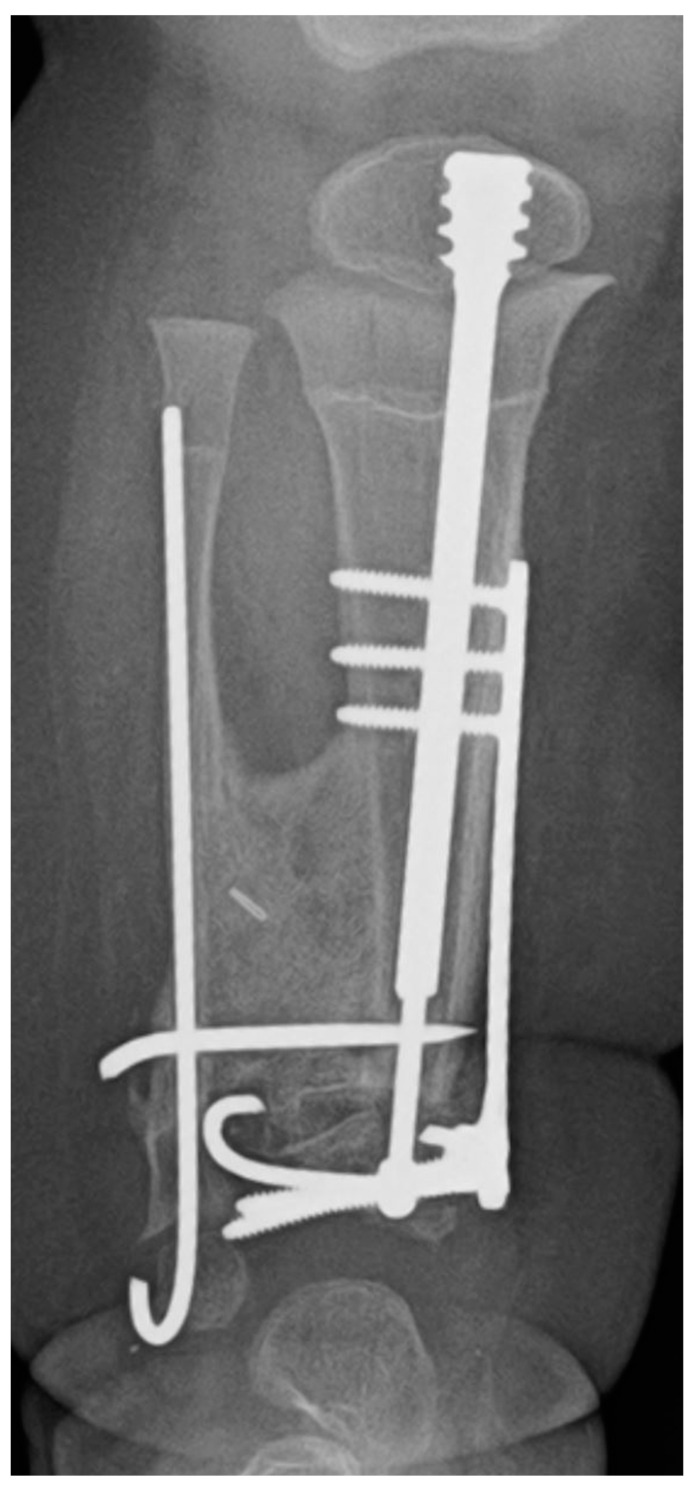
AP radiograph showing an example of a very distal pseudarthrosis site. This required fixation into the distal tibial epiphysis as there was not enough distal tibial metaphysis for screw placement. The cross-union is fully healed, and these screws can now be removed to allow the physis to resume growth.
